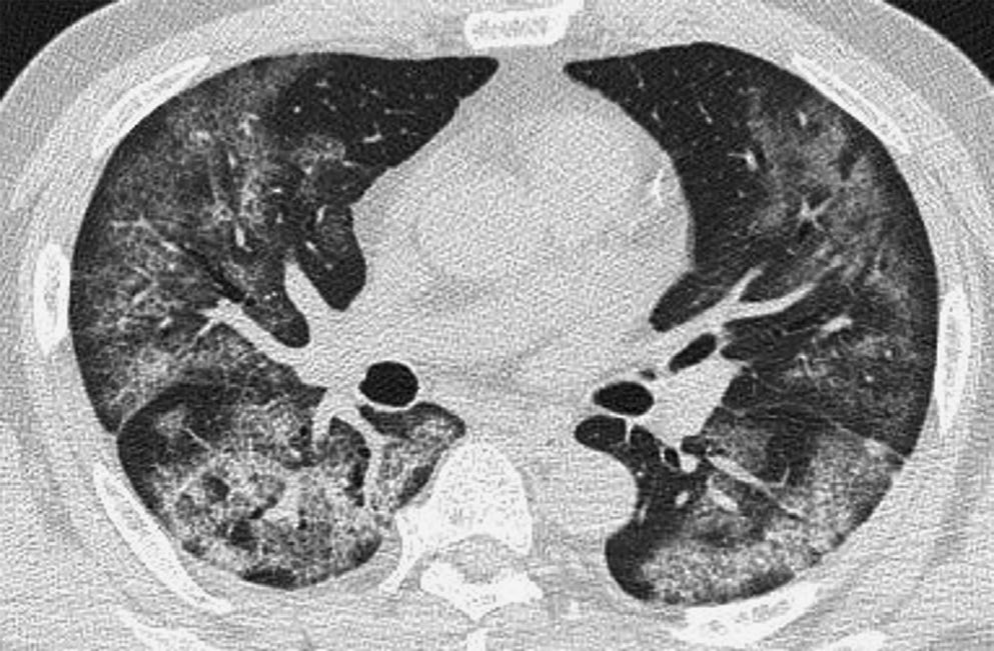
Chest computed tomography showing bilateral pulmonary crazy paving appearances
